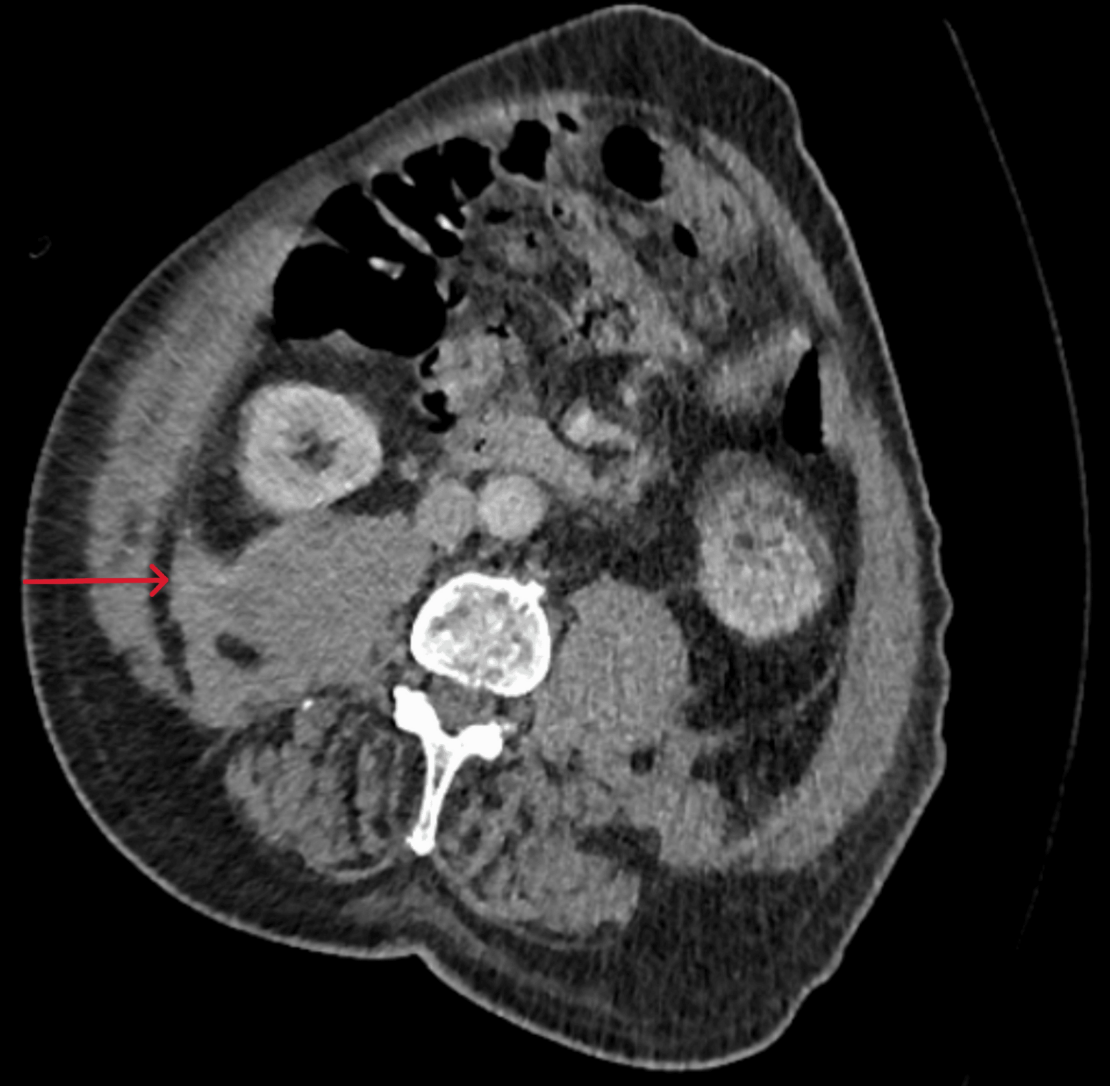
CT abdomen showing high-density fluid along the right psoas muscle, suggestive of hematoma (red arrow).
